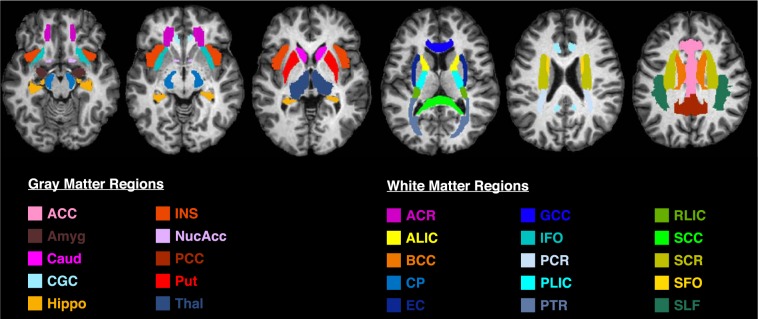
T1-weighted axial MPRAGE image with 25 regions of interest obtained using the JHU_MNI_SS (‘Eve’) atlas. Colors indicate different brain structures. The 15 white matter structures include - ACR: Anterior Corona Radiata, SCR: Superior Corona Radiata, CP: Cerebral Peduncle, PLIC: Posterior Limb of Internal Capsule, ALIC: Anterior Limb of Internal Capsule, RLIC: Retrolenticular part of Internal Capsule, PTR: Posterior Thalamic Radiation, PCR: Posterior Corona Radiata, IFO: Inferior Fronto-Occipital Fasciculus, SFO: Superior Fronto-Occipital Fasciculus, SLF: Superior Longitudinal Fasciculus, BCC: Body of Corpus Callosum, EC: External Capsule, GCC: Genu of Corpus Callosum, SCC: Splenium of Corpus Callosum. The 10 grey matter structures include - ACC: Anterior Cingulate Cortex, PCC: Posterior Cingulate Cortex, Caud: Caudate nucleus, CGC: Cingulate Gyrus (Cortex), INS: Insular cortex, Thal: Thalamus, Put: Putamen, Hippo: Hippocampus, Amyg: Amygdala, and NuAcc: Nucleus Accumbens.
